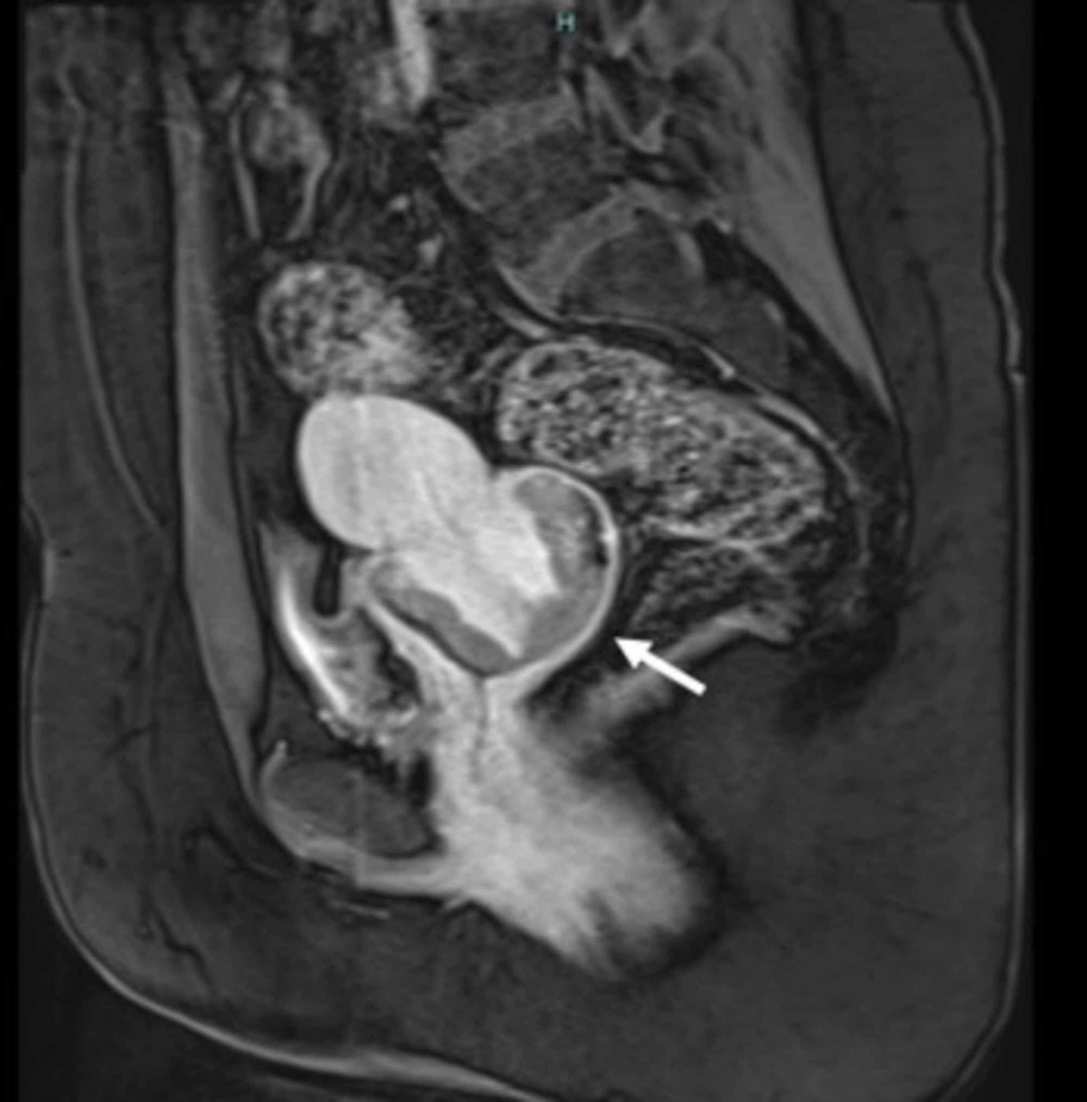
Pelvic MRI before treatment, showing the cervical mass projecting into the vagina
